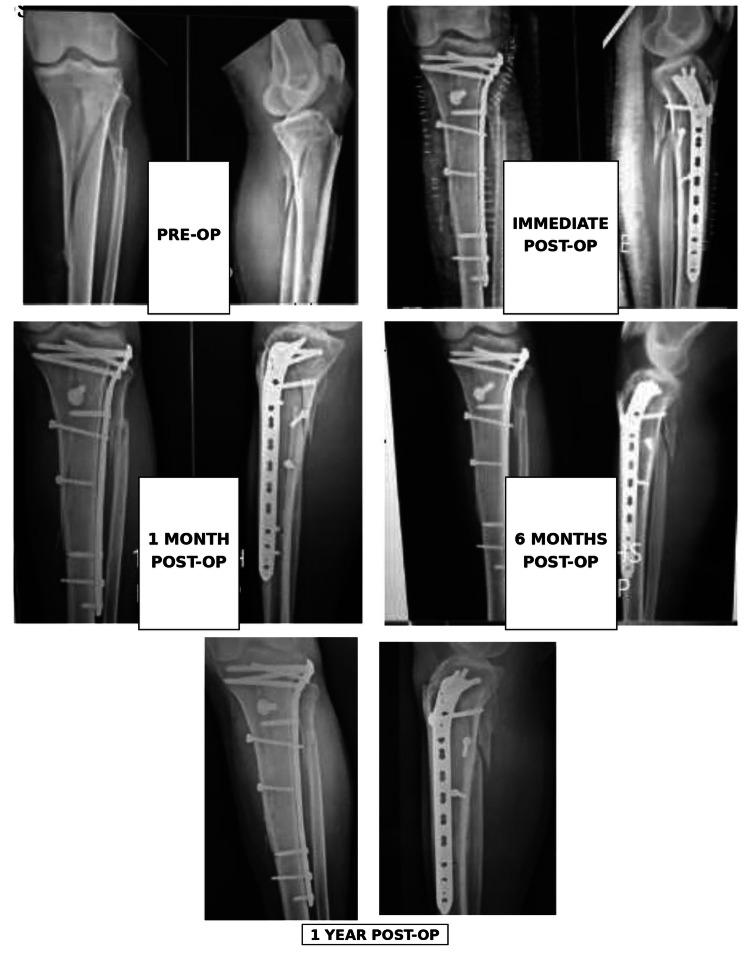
Representative MIPPO case showing radiographic progression Top row: preoperative radiographs and immediate postoperative radiographs after submuscular locking compression plate fixation. Middle row: one-month and six-month follow-up showing progressive callus formation. Bottom row: 12-month radiographs (anteroposterior and lateral) demonstrating complete radiological union with maintained axial alignment MIPPO: minimally invasive percutaneous plate osteosynthesis
